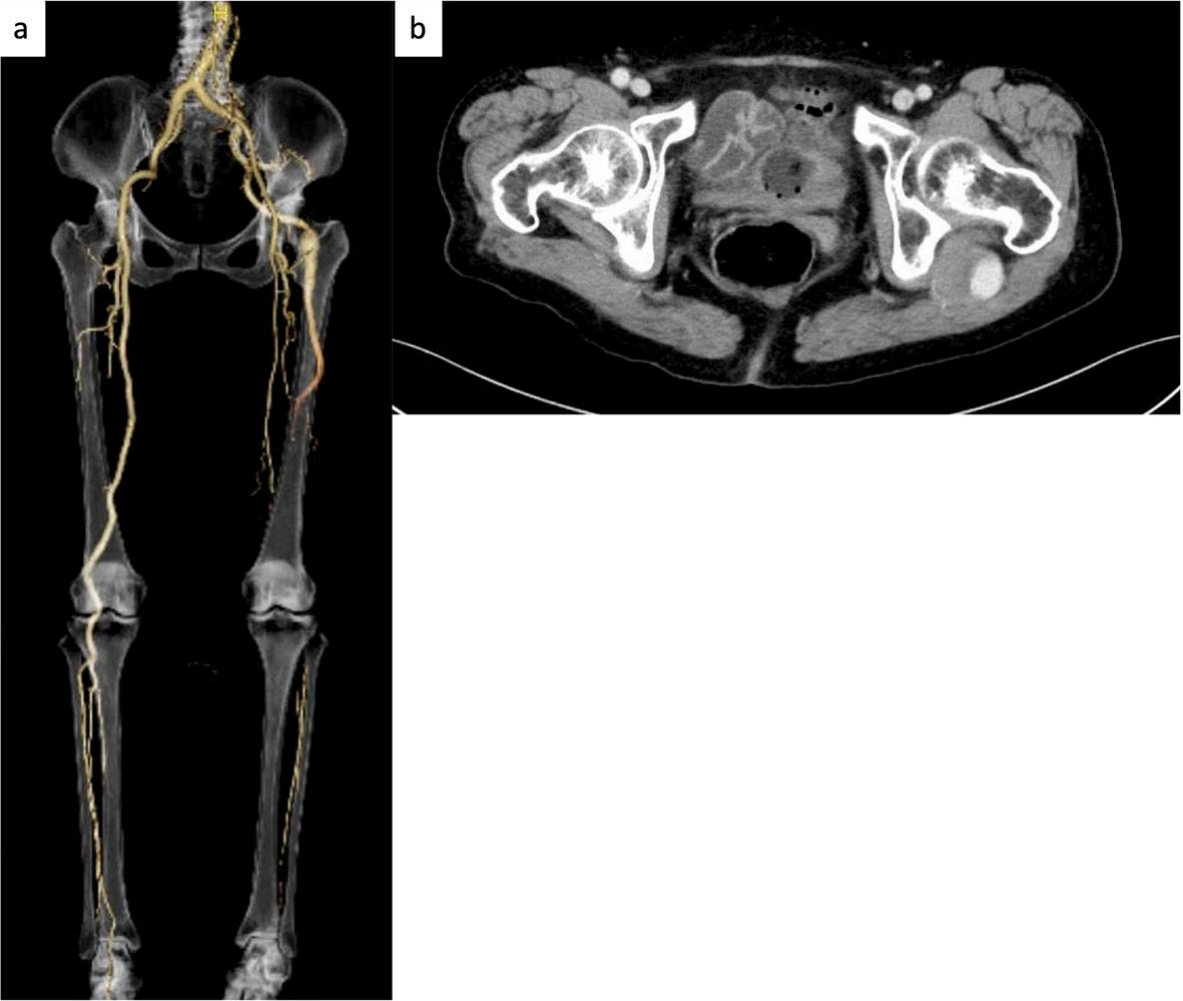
a, b Enhanced computed tomography angiography revealing a left PSA aneurysm and enhanced collateral flow toward the occluded PA below the knee joint distal to a hypoplastic, incomplete SFA. PSA, persistent sciatic artery; PA, popliteal artery; SFA
